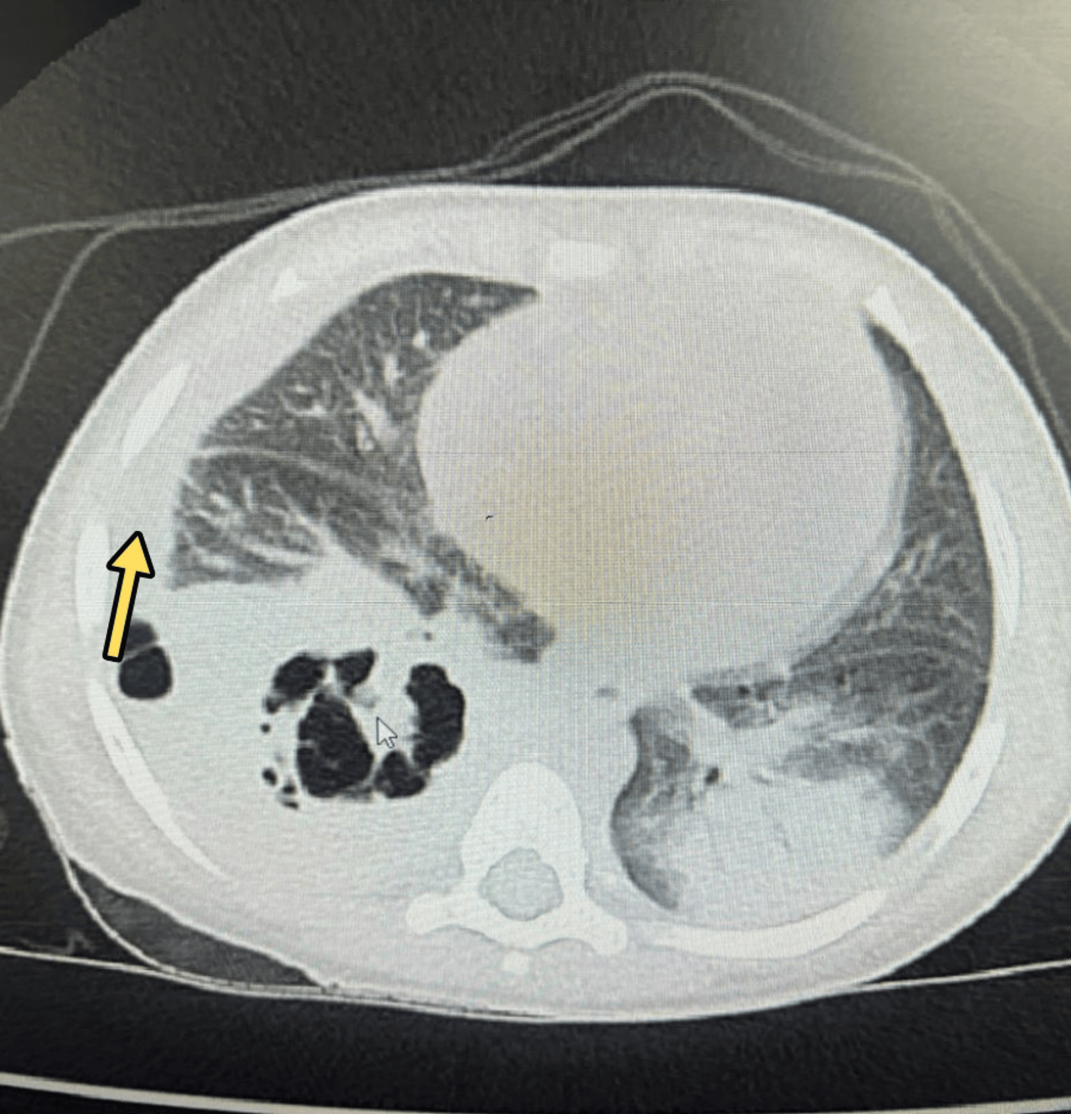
Right pleural effusion Contrast-enhanced computed tomography of the chest showing dense consolidation in both lower lobes with right pleural effusion (yellow arrow).
